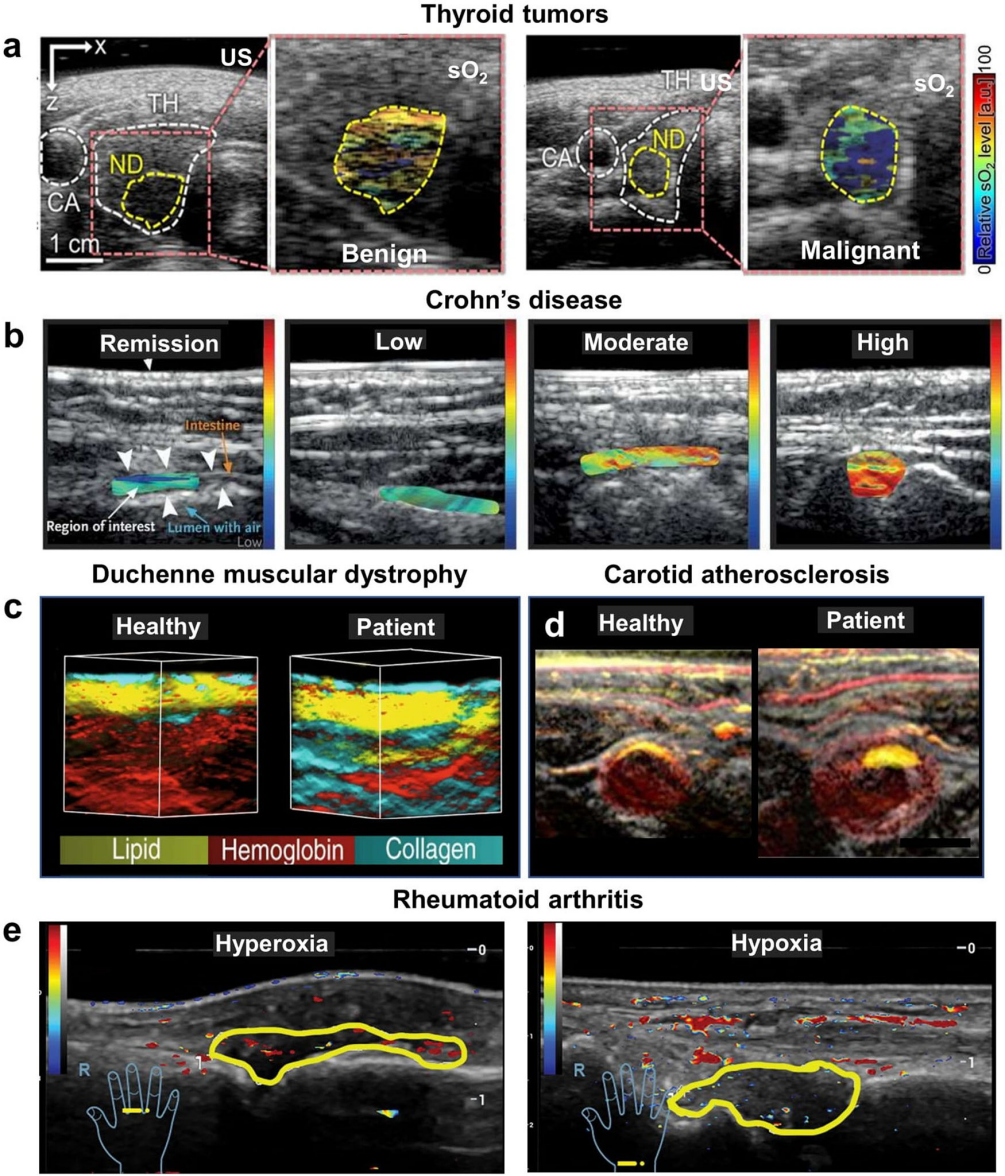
Representative in vivo macroscopy images of healthy volunteers or patients with different diseases. a Ultrasound (US) and co-registered optoacoustic (OptA)/US images showing hemoglobin oxygen saturation (sO_2_) in patients with a benign nodule and papillary thyroid cancer (PTC). CA carotid artery, ND nodule, TH thyroid. Adapted from Kim et al.^43^ with permission from AACR. b Co-registered US and OptA images showing total hemoglobin in the intestines of Crohn’s disease patients with different severity or remission. Adapted from Knieling et al.^47^. Copyright © (2017) Massachusetts Medical Society. Reprinted with permission from Massachusetts Medical Society. c 3D multispectral optoacoustic tomography (MSOT) images of a healthy volunteer and a patient with Duchenne muscular dystrophy. Adapted with permission from Regensburger et al.^87^. d Merged US/MSOT images of the carotid artery region reconstructed using prior-integrated reconstruction from a healthy volunteer and a patient with carotid atherosclerosis. Red: 850 nm, Yellow: 930 nm. Scale bar: 1 cm. Adapted with permission from Yang et al.^54^. e Oxygen saturation images of patients with rheumatoid arthritis reporting hyperoxia (red) and hypoxia (blue). Used with permission of Radiological Society of North America from Yang et al.^79^; permission conveyed through Copyright Clearance Center, Inc.
